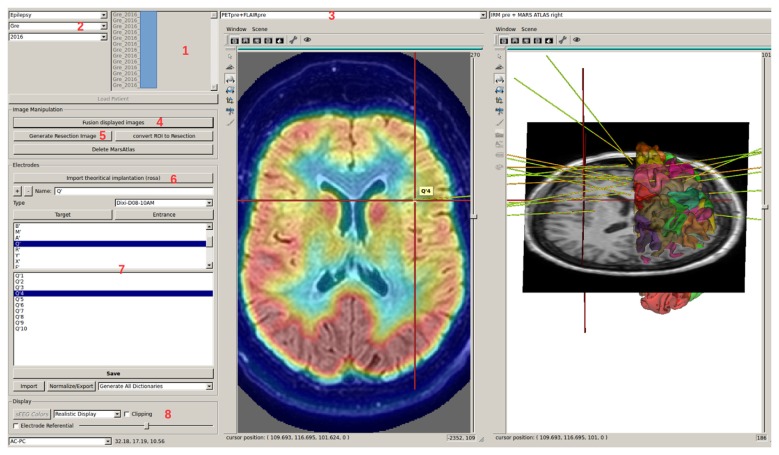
LocateElectrodes interface allows to select a patient in the database (1), using searching criteria (2). The user can navigate in the different imported images using (3), fusion them easily (4), generate the resection mask (5) if the T1 post-resection was imported. Using (6), the user can add, position and name electrodes after choosing a predefined 3D model. Clicking on electrode/contact names (7) updates the 3D Anatomist viewers on the right (here on contact Q’4), and the red 3D cursor moves to the contact. The view can be axial/sagittal/coronal, or in the electrode referential using (8). Here, Positron Emission Tomography (PET) and T1 MRI fusion are displayed on the left, MarsAtlas cortical parcellation and T1 MRI on the right with the implanted electrode models.
